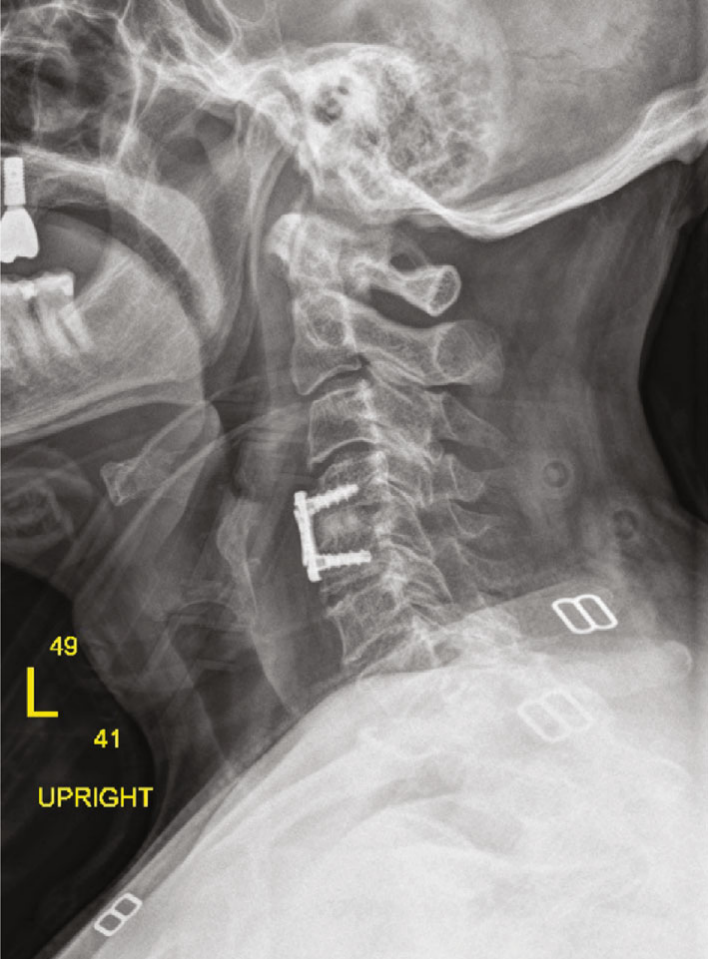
Lateral radiograph obtained six weeks postoperatively. Lateral radiograph reveals status-postanterior cervical discectomy with placement of allograft spacer and anterior cervical plate with variable angle screws.
